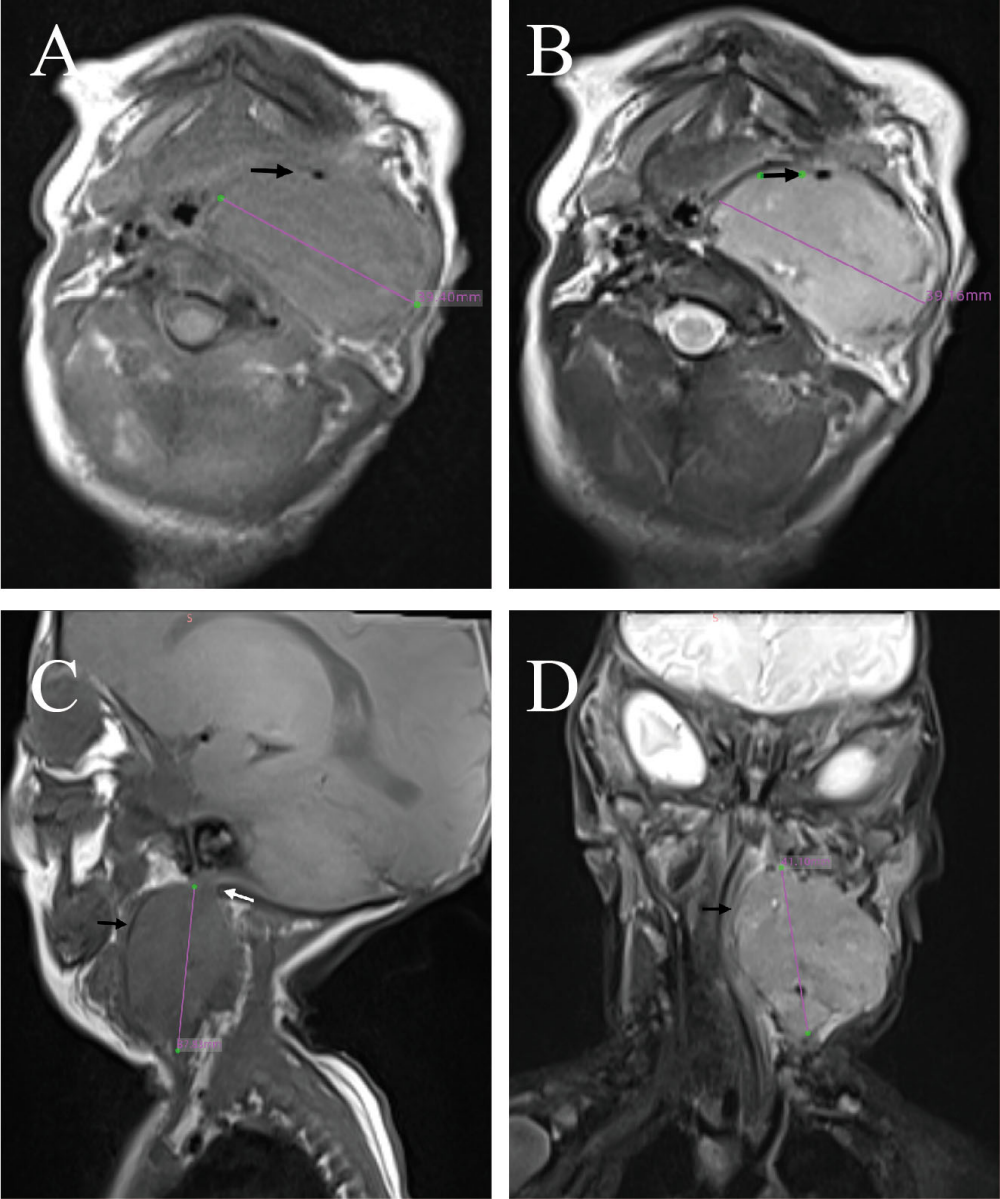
MRI of case 2. (A) T1 transverse section. (B) T2 transverse section. (C) T2 sagittal section. (D) T2 coronal section. (A, B) The tumor is encapsulated with a well-defined border. The black arrows indicate the internal carotid artery, with the external carotid artery and internal jugular vein showing occlusion. There is partial liquefactive necrosis in the center of the tumor. (C) Superior aspect of the tumor showing an indistinct boundary from the skull base tissues. (D) The tumor compressing into the laryngeal cavity.
